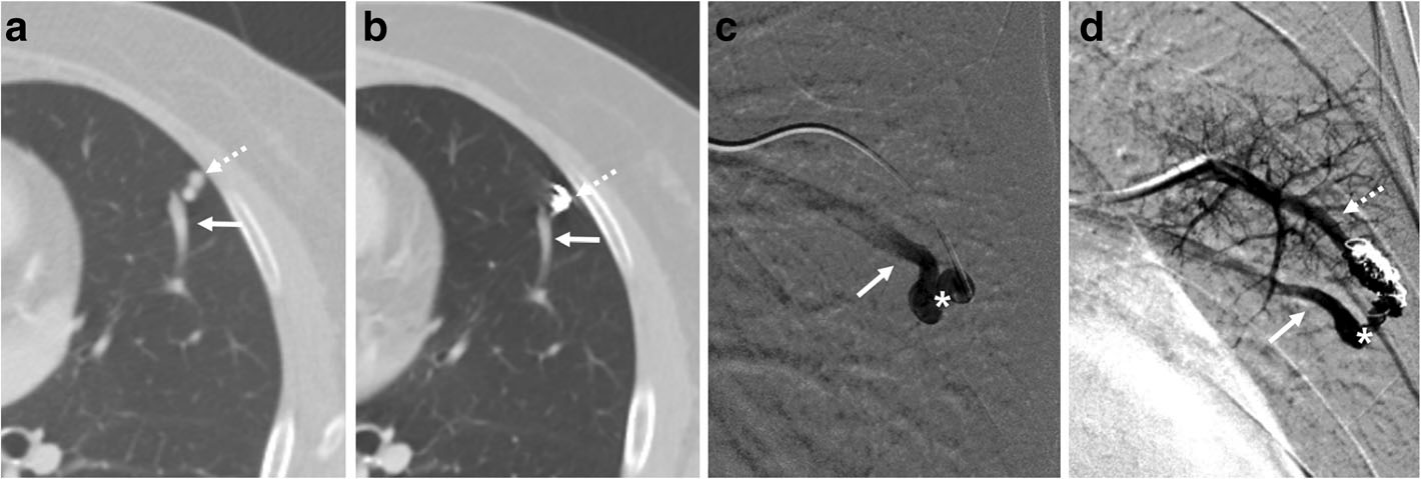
A 56-year-old female who underwent embolization 17 months earlier with simple PAVM of the left upper lobe. Arrows, dashed arrows, and asterisks indicate draining vein, feeding artery, and venous sac, respectively. CT images obtained before (a) and after (b) embolization. The draining vein decreased from 3.59 mm to 3.28 mm after embolization (8.6 % reduction) and coils were deployed in the feeding artery. Recanalization was confirmed by pulmonary angiography obtained before (c) and after (d) embolization
